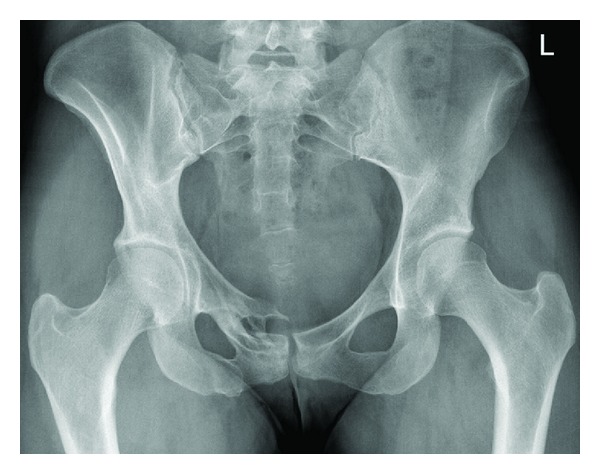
Anteroposterior radiographs of the pelvis demonstrating the right superior pubic fracture 5 years after the RTA.
